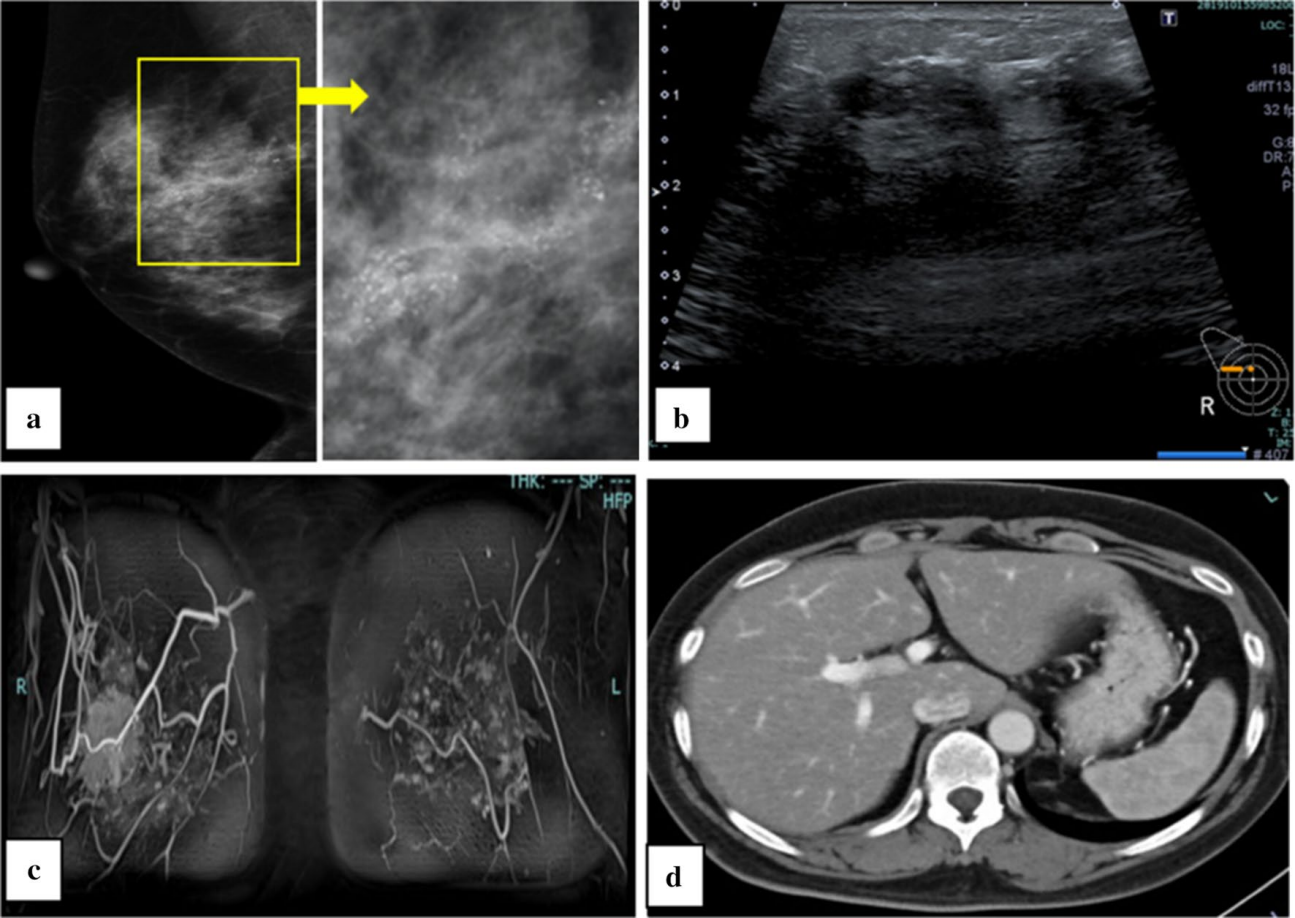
Imaging findings. a Mammogram shows segmental polymorphic calcifications (yellow square) in the right ML-UM (BI-RADS 5). b Ultrasound revealed an axial 45 mm hypoechoic mass with internal coarse calcifications in the right breast (BI-RADS 5). c Contrast magnetic resonance imaging showed wide-range non-mass enhancement in the upper-outer quadrant of the right breast. d Contrast computed tomography showed that there was no distant metastasis and liver morphology was maintained and no gallstones were noted
